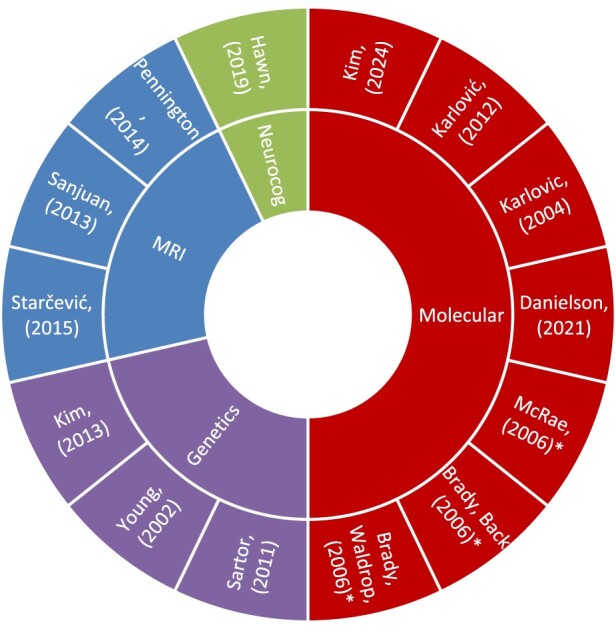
Sunburst chart. The proportion of studies using each biomarker domain is shown in the inner ring. *Same participant sample. Neurocog = neurocognition; MRI = magnetic resonance imaging.
